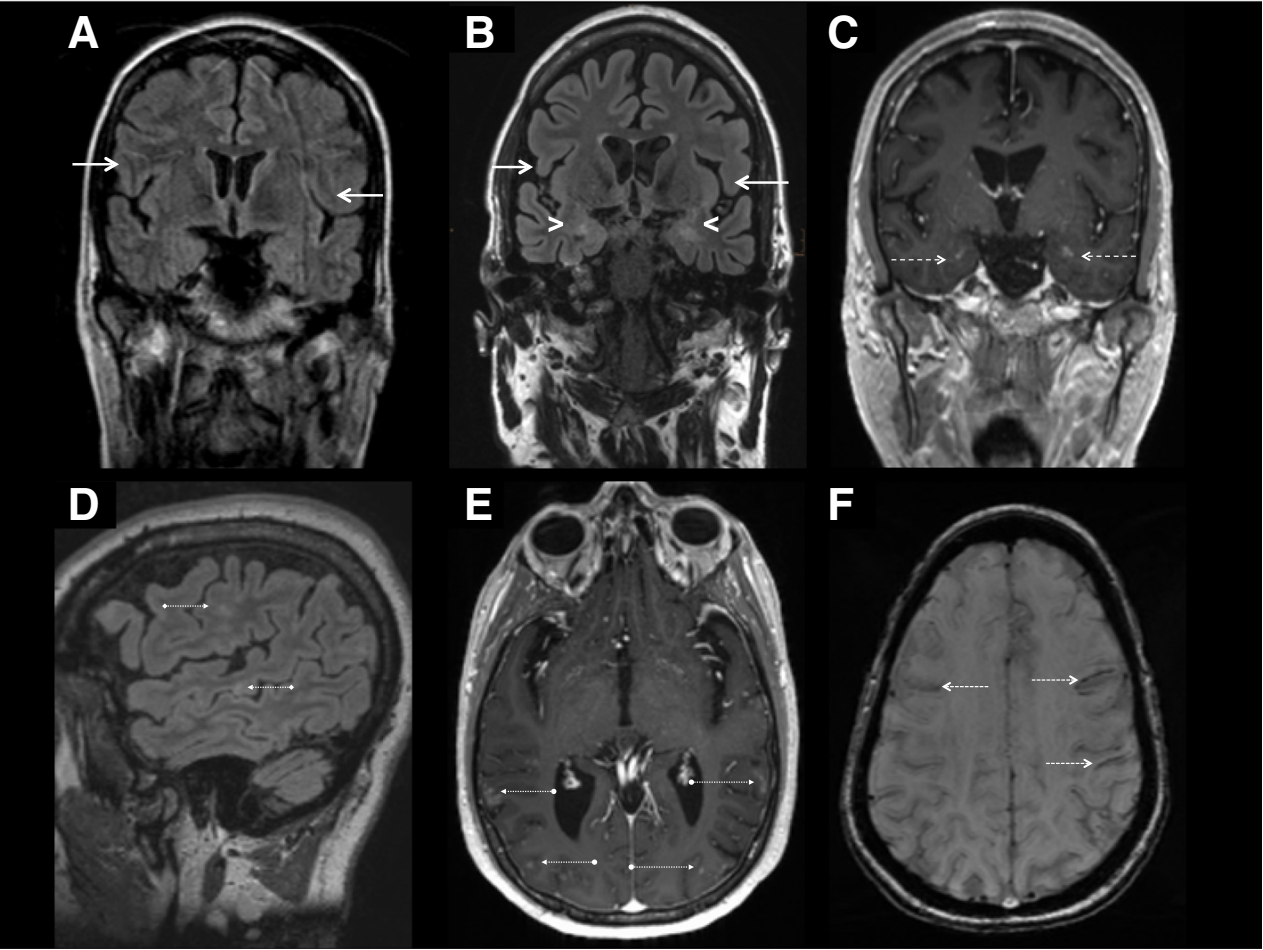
MRI-imaging reveals progressive atrophy of the temporal structures (a, b) and a new signal increase of the corpora amygdaloidea and hippocampi in FLAIR weighted sequences (c) over the course of time. Several spots of cortical hyperintensities are present in FLAIR weighted imaging (d). A faint contrast enhancement can be detected in the bihemsipheric cortex (e) and the corpora amygdaloidea (c). Mesencephalic and cortical hemorrhage (f) suggest an inflammatory process with microvascular vasculitis or an infectious or granulomatous genesis as possible differential diagnoses
